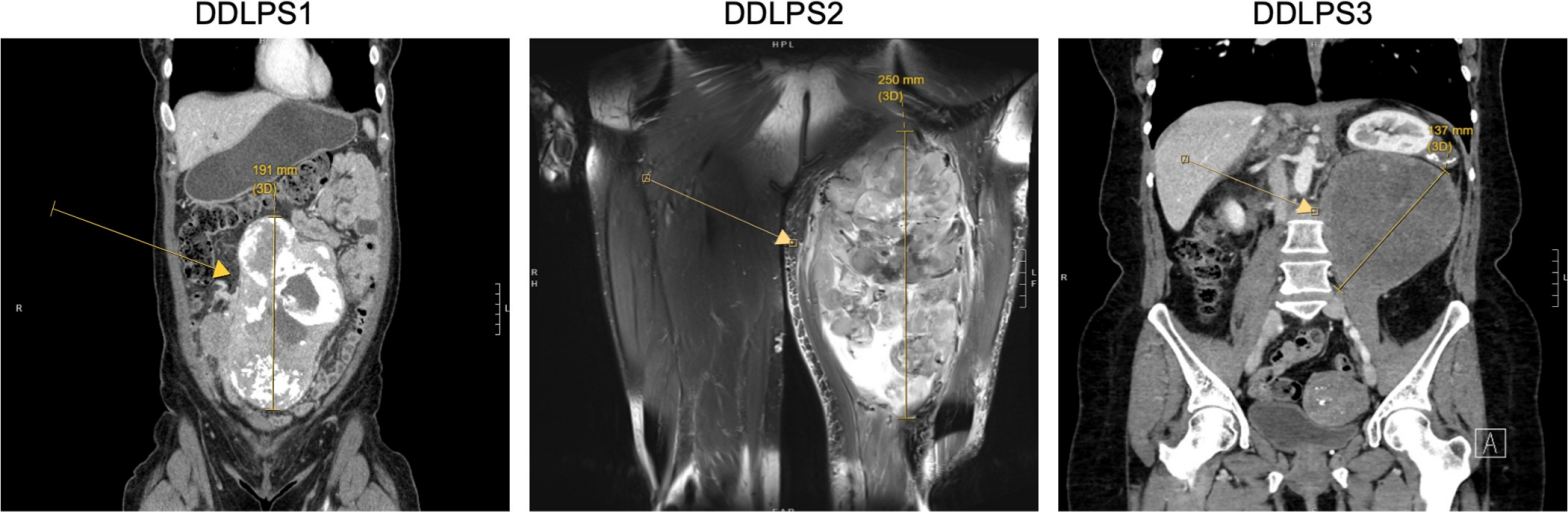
Computed tomography scans (DDLPS1 and DDLPS3) and a T2 fat-suppressed magnetic resonance image (DDLPS2) of patients with DDLPS. The tumor of patient DDLPS3 was mostly well differentiated liposarcoma, which is reflected on imaging by the low density, homogenous appearance.
